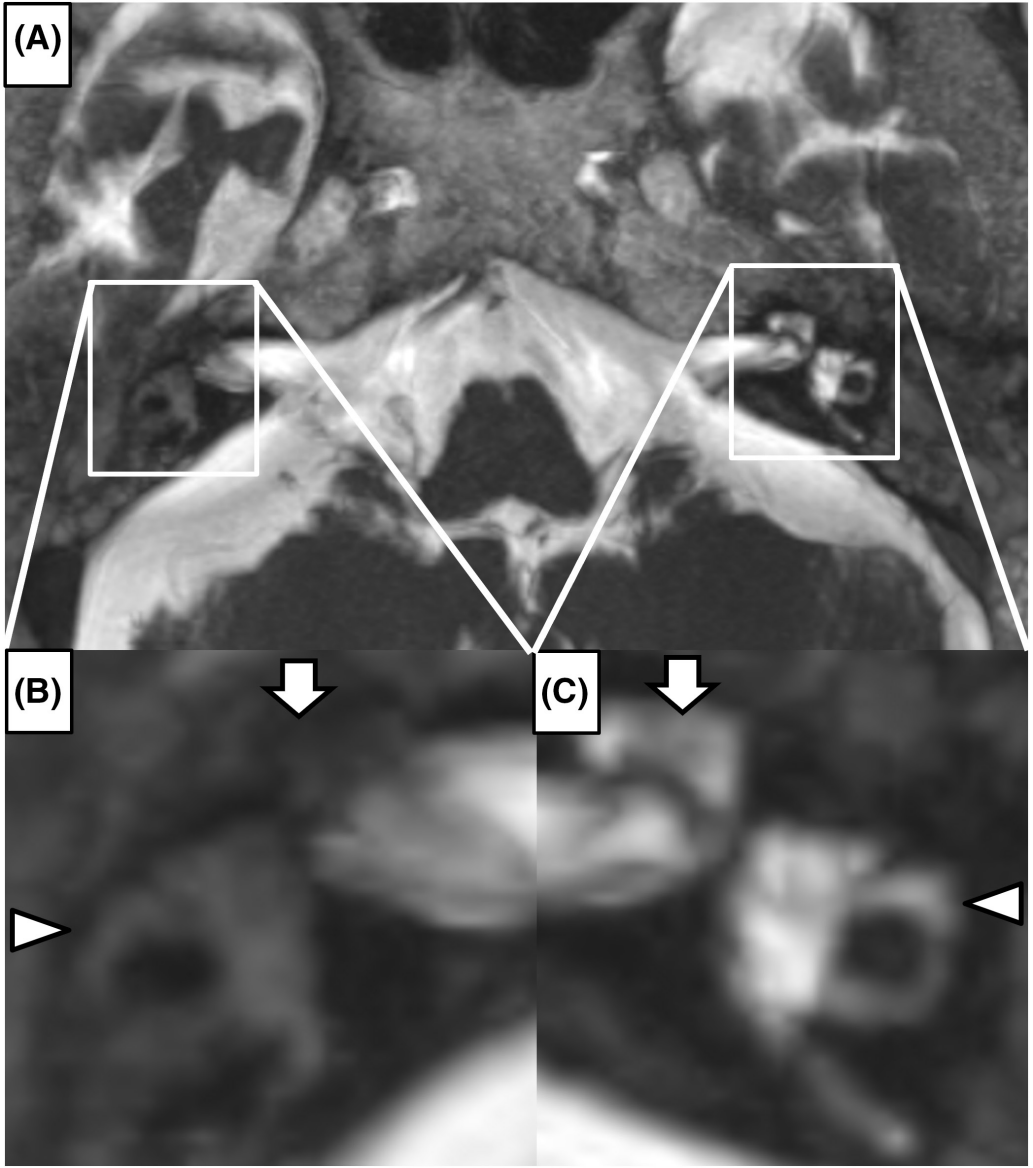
(A) MRI of T2‐weighted image showed decreased signal intensity in the right inner ear, demonstrating the process of inner ear ossification. (B) Detailed view of the right inner ear; (C) detailed view of the left inner ear (arrow: cochlear; arrowhead: semicircular canal)
